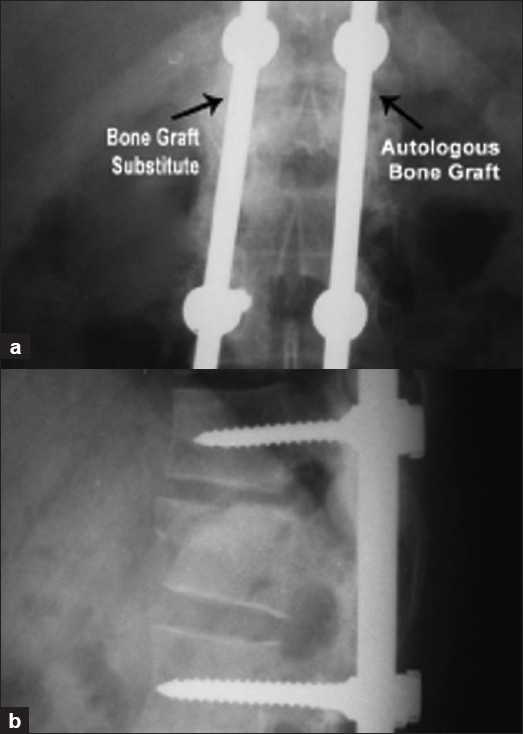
One year follow up X-ray [anteroposterior (a) and lateral view (b)] of the same patient showing bridging bone mass with graft incorporation on both the sides
